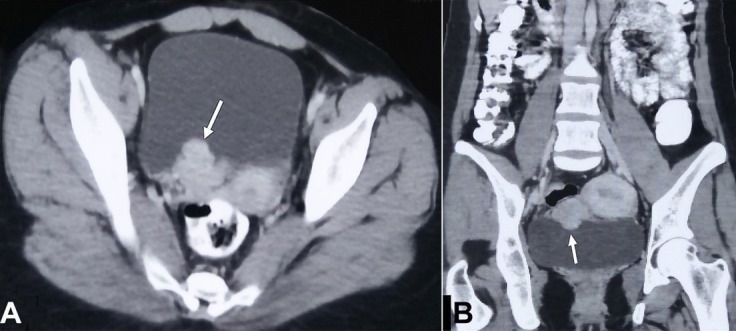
A – Axial; and B – Coronal contrast enhanced CT abdomen reveal nodular, polypoidal enhancing mass (white arrow) arising from right posterior wall of urinary bladder.
